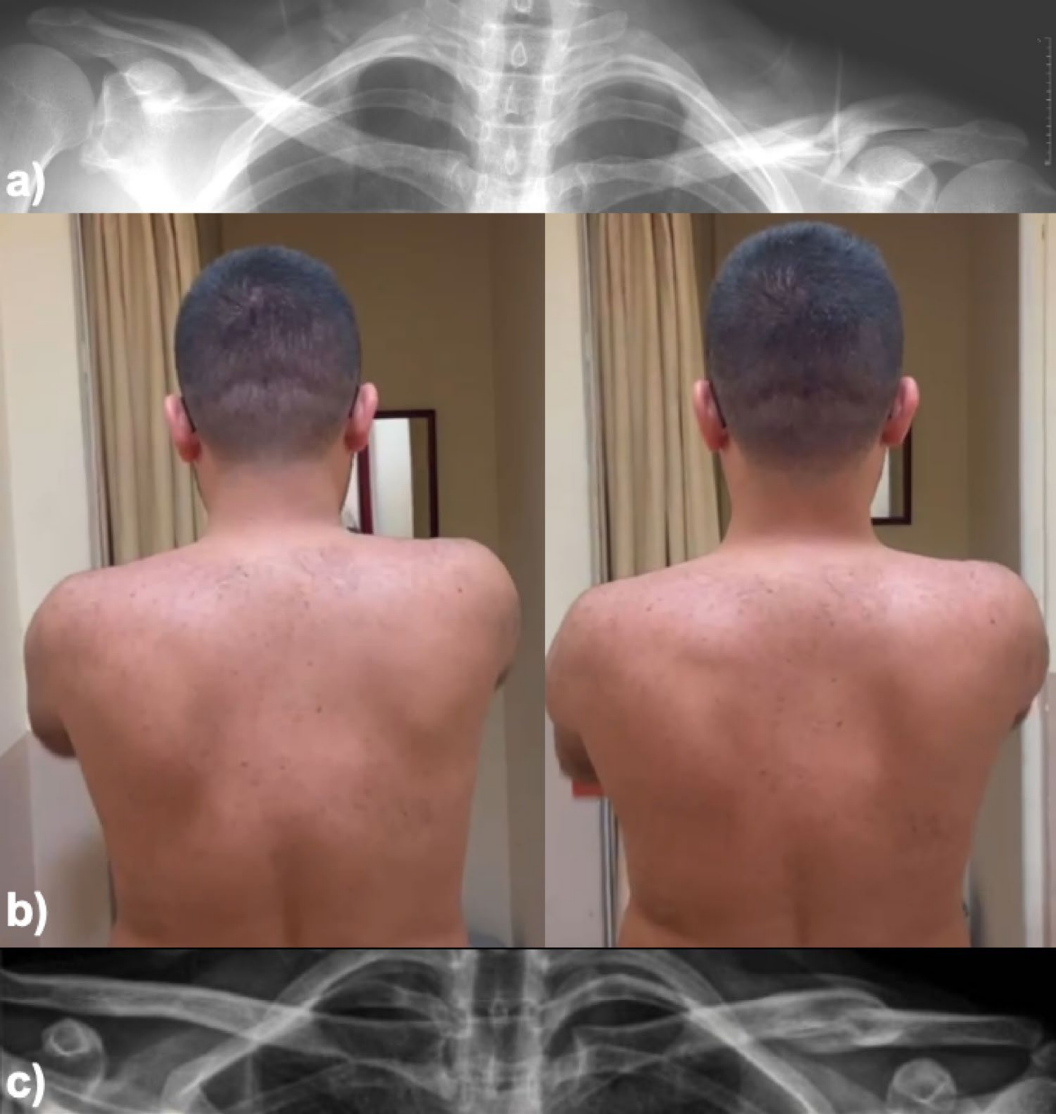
Radiograph of a 30-year-old male patient who presented with left clavicle shaft fracture after a motor vehicle accident (a), clinical photograph and X-ray image of a patient who presented with left scapular dyskinesis after 4 years of follow-up following conservative treatment (b, c)
